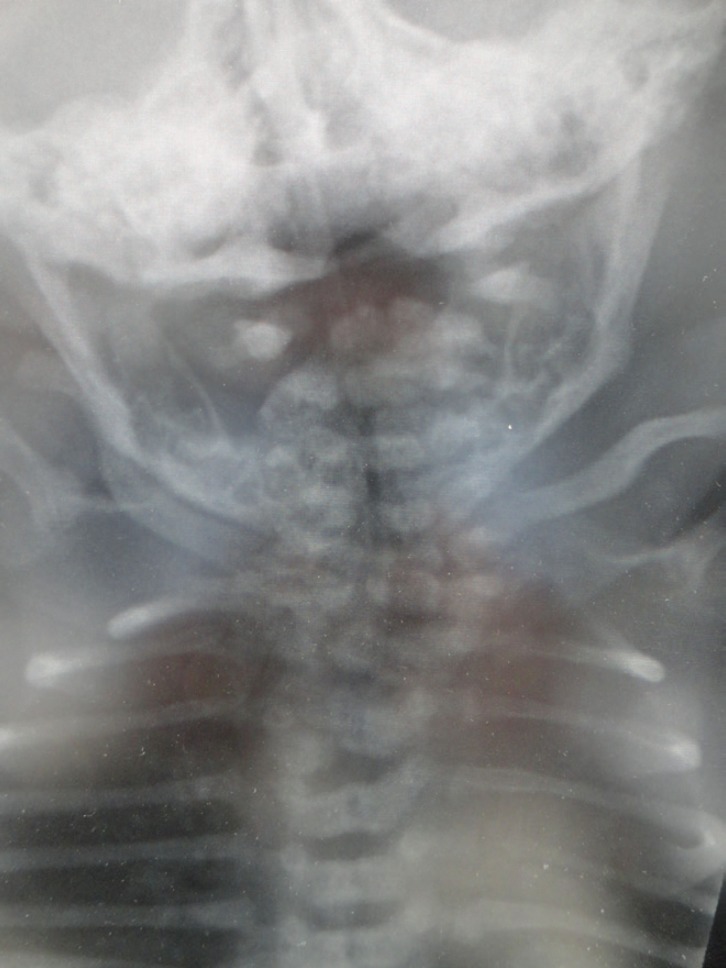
Neck radiograph showing vertebral segmentation defect involving cervical and upper thoracic vertebrae
